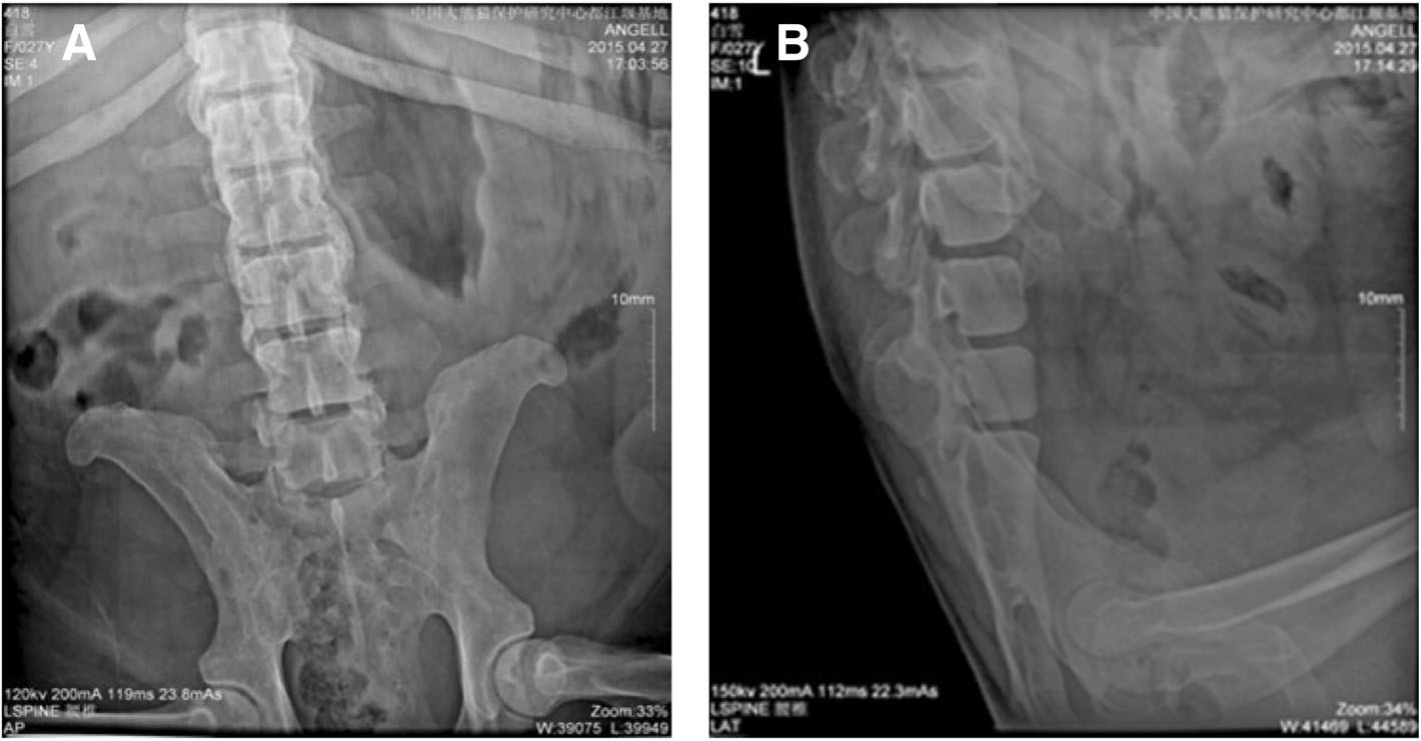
The results of Digital Radiography diagnosis. The lateral longitudinal ligament and anterior longitudinal ligament of the lumbar occurred band calcification with the formation of a bridge of the bone. a Lumbar anteroposterior position. b Lumbar lateral position
